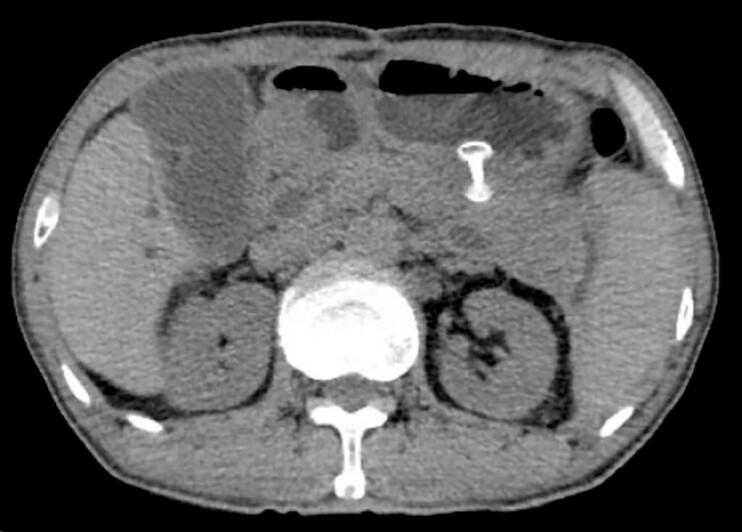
Postoperative abdominal computed tomography shows the resolution of pseudocyst and pancreatic duct dilation.
